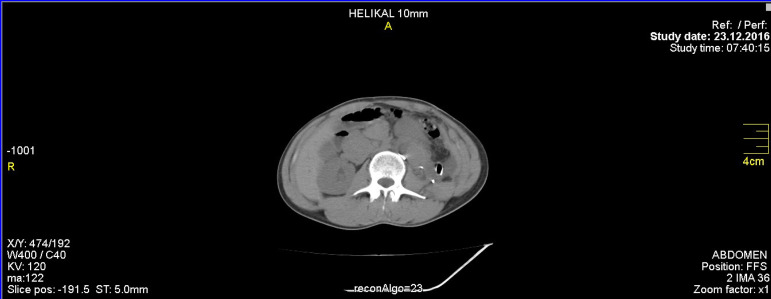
Thoracoabdominal computed tomography before discharge.
